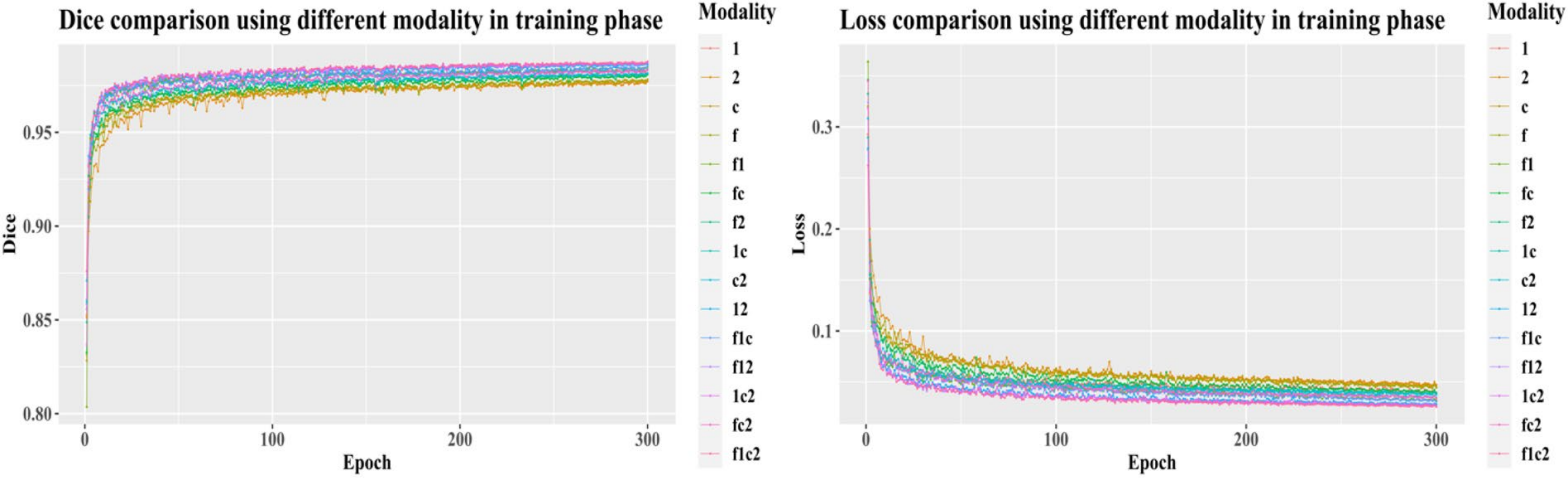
The change of dice (left) and loss (right) in the training phase. In the legend, 1, 2, c, and f represent T1, T2, T1ce, and T2-FLAIR, respectively. For example, f1ce represents that the combination has all four image sequences.
